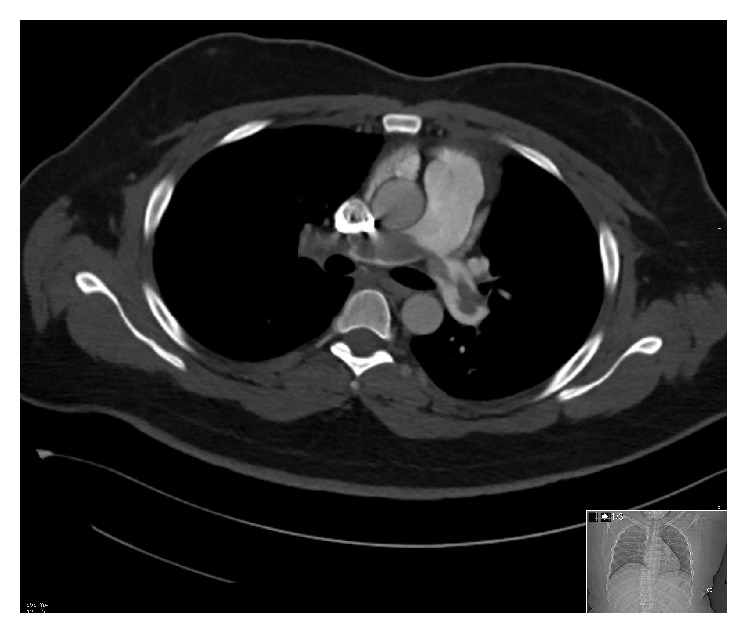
CT pulmonary angiogram revealed a saddle embolus extending into the main, lobar, and segmental pulmonary arterial branches bilaterally with massive clot burden and near complete occlusion of the right main and branch pulmonary arteries.
